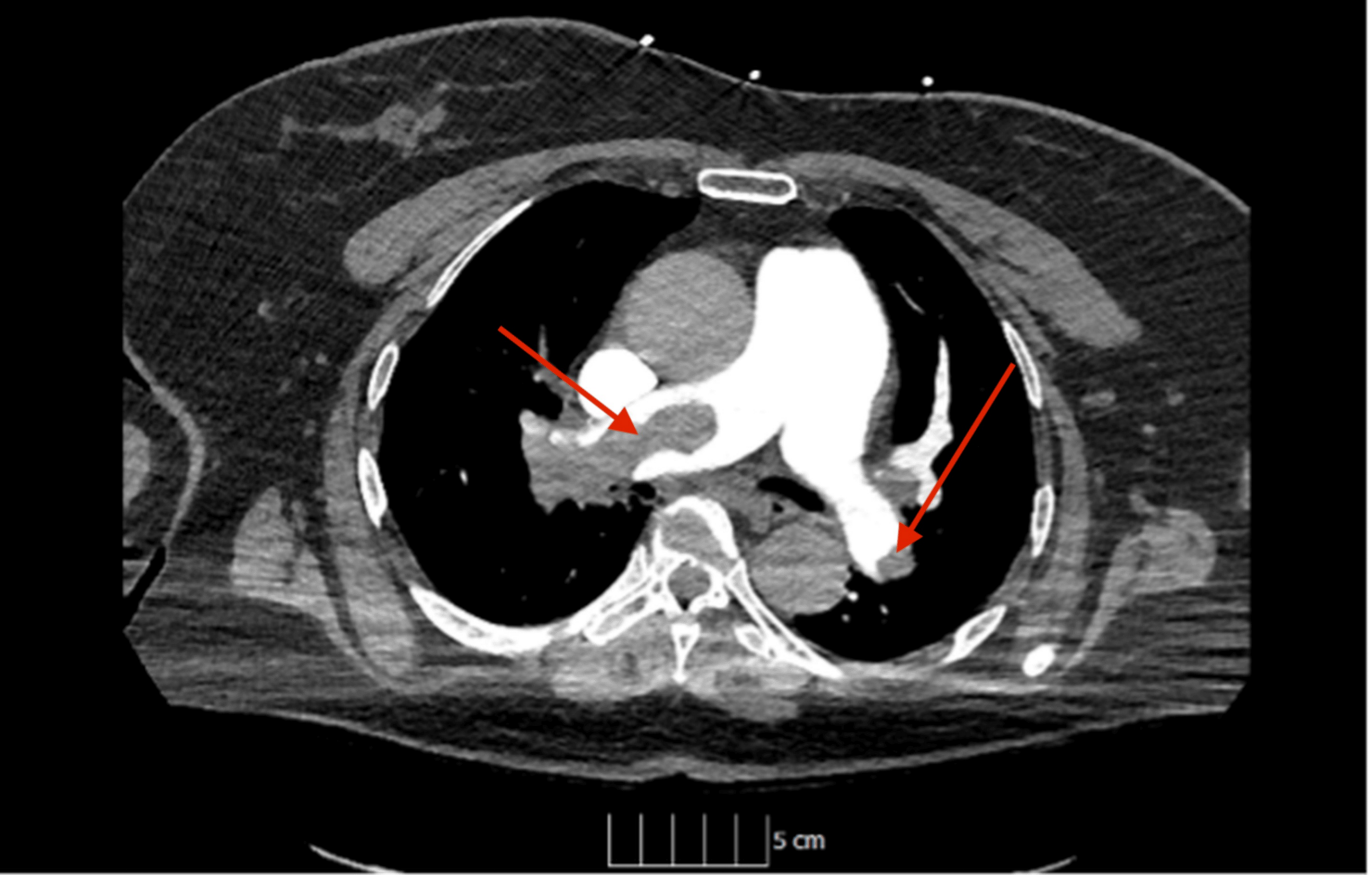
Axial images of a computed tomography pulmonary angiogram Showing bilateral pulmonary embolism with occlusive thrombus in the right main pulmonary artery (left arrow) and likely non-occlusive in the left main pulmonary artery (right arrow).
